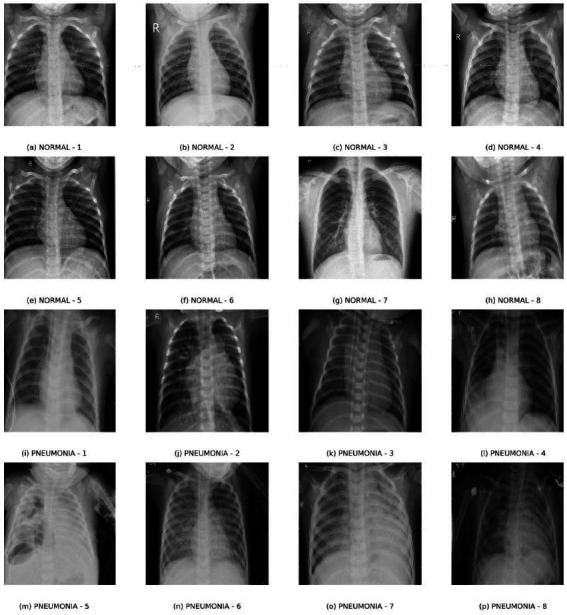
Sample input images from the “Chest X-ray images (pneumonia)” dataset.
